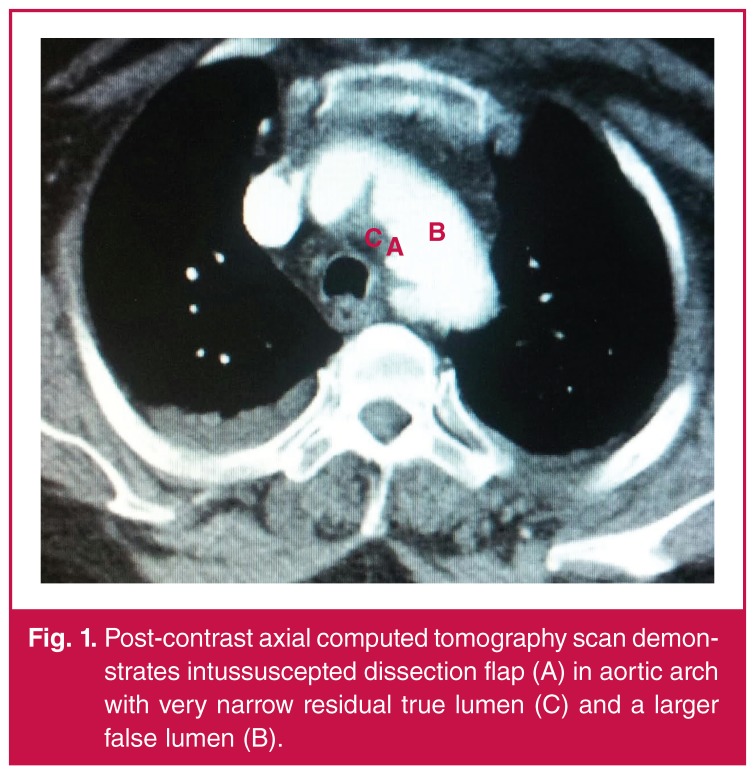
Post-contrast axial computed tomography scan demonstrates intussuscepted dissection flap (A) in aortic arch with very narrow residual true lumen (C) and a larger false lumen (B).
